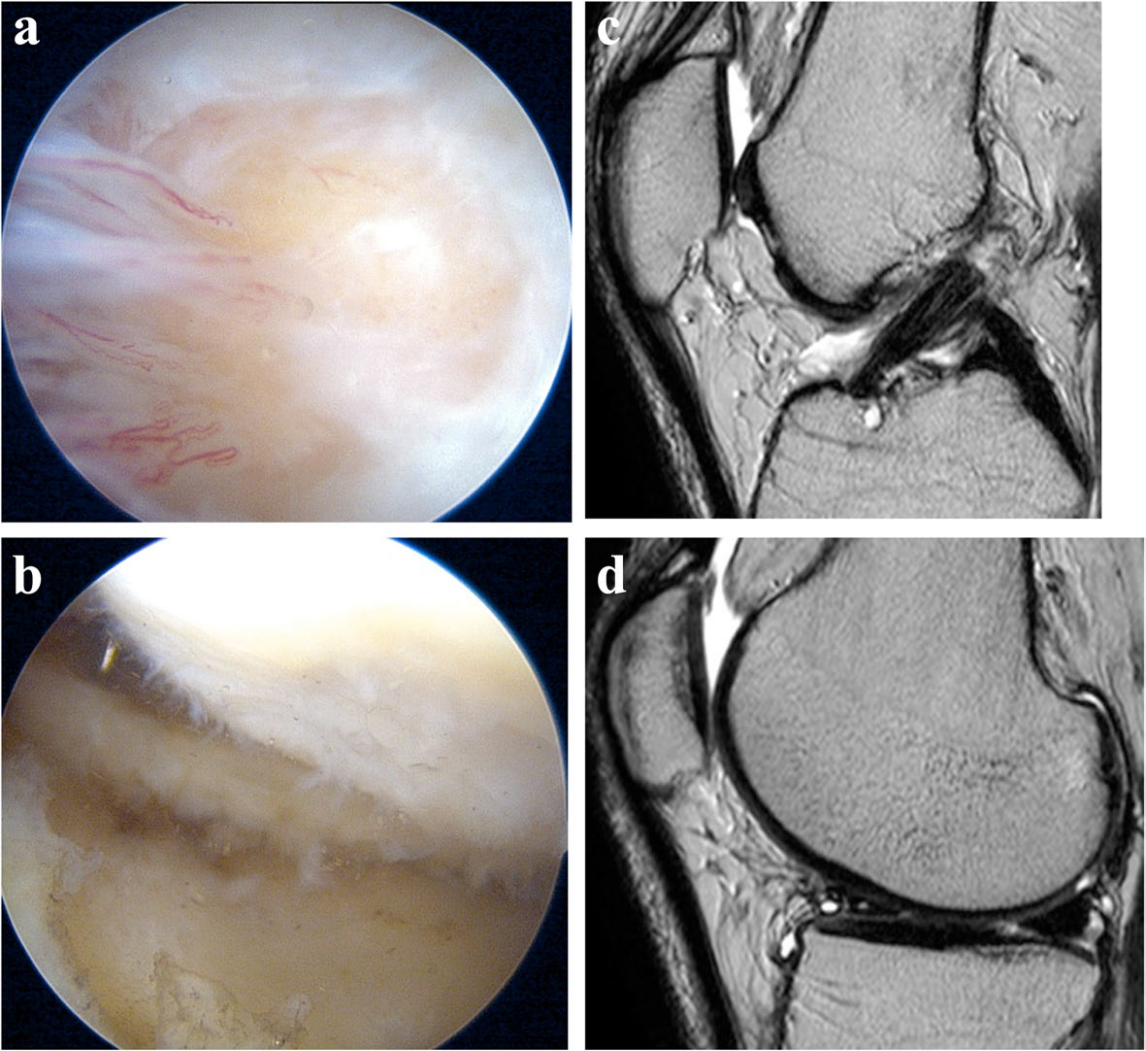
The oversight in the preoperative MRI case. a The arthroscopy showed 11 × 18 mm grade 4 cartilage injury in the trochlea. b The arthroscopy showed 10 × 10 mm grade 3 cartilage injury in the lateral femoral condyle. c His preoperative MRI showed the unevenness of articular cartilage with associated effusions in T2-weighted sequences in the trochlea. d His preoperative MRI showed the unevenness of articular cartilage with associated effusions in T2-weighted sequences in the lateral femoral condyle
